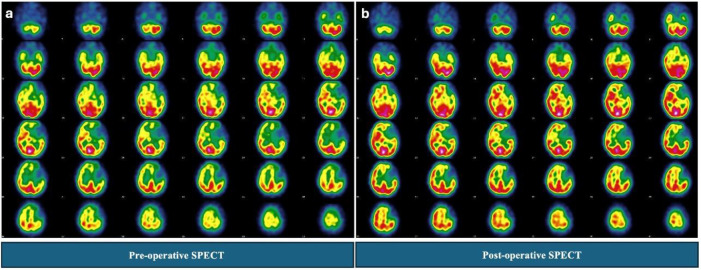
The 29 year old patient had extensive Type III CVR responses bilaterally in the frontal and parietal regions (predominantly left sided) before surgery (a). Postoperatively, the patient developed new perfusion deficits. Follow-up SPECT confirmed these new areas of hypoperfusion, which were predominantly located in regions that had demonstrated the most severe preoperative Type III responses (b)
